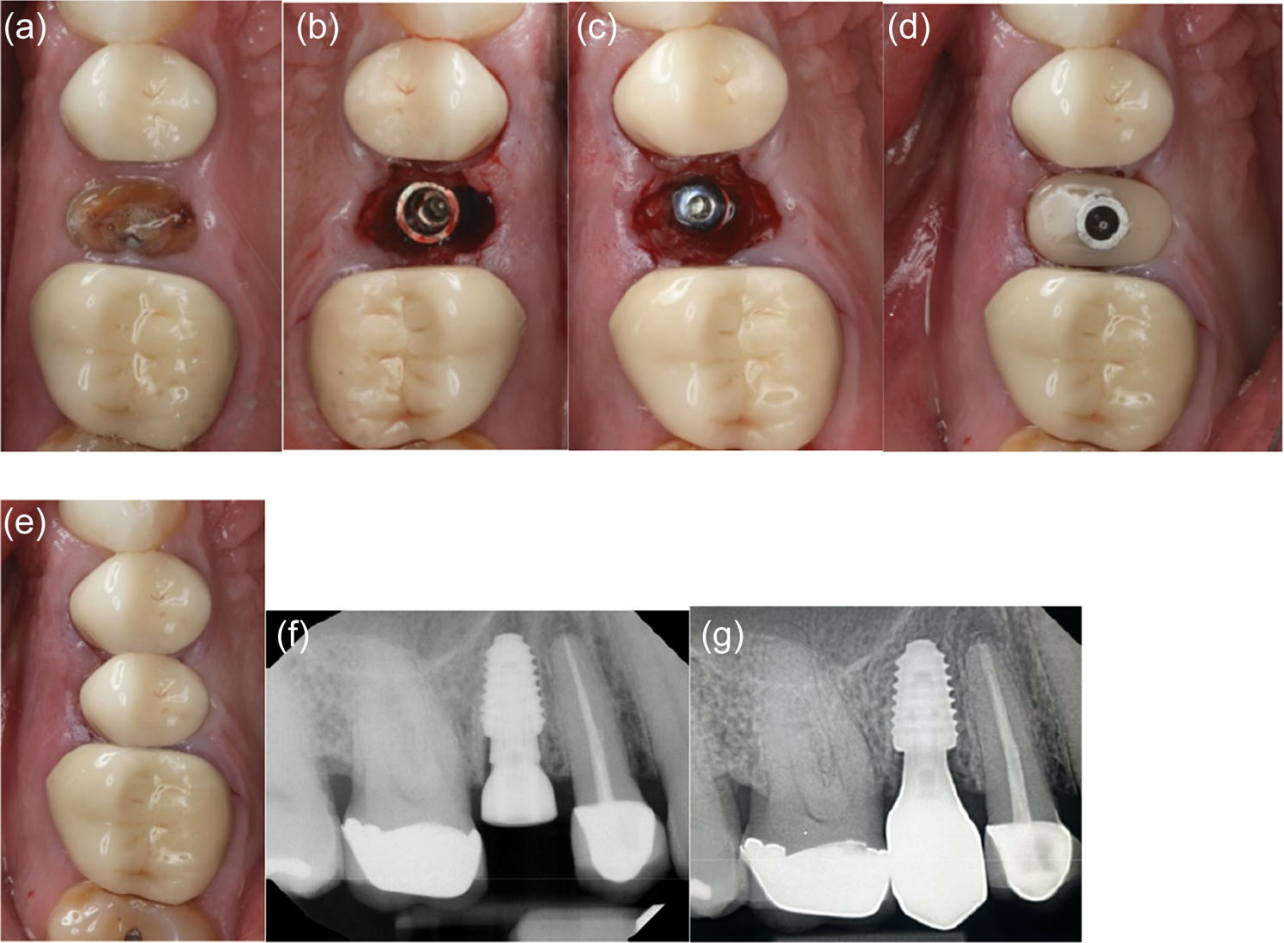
Minimally invasive removal of fractured premolar and placement of immediate implant without osteotomy on Patient 3: (a) Fractured non‐restorable premolar. (b) Prepless immediate implant. (c) Cover screw placed to accommodate grafting with cortical cancellous allograft. (d) Anatomical healing abutment placed on the day of surgery. (e) Final monolithic Zr restoration. (f) Peri‐apical radiograph of day of surgery with anatomical healing abutment. (g) Peri‐apical radiograph with definitive restoration at 12 months.
